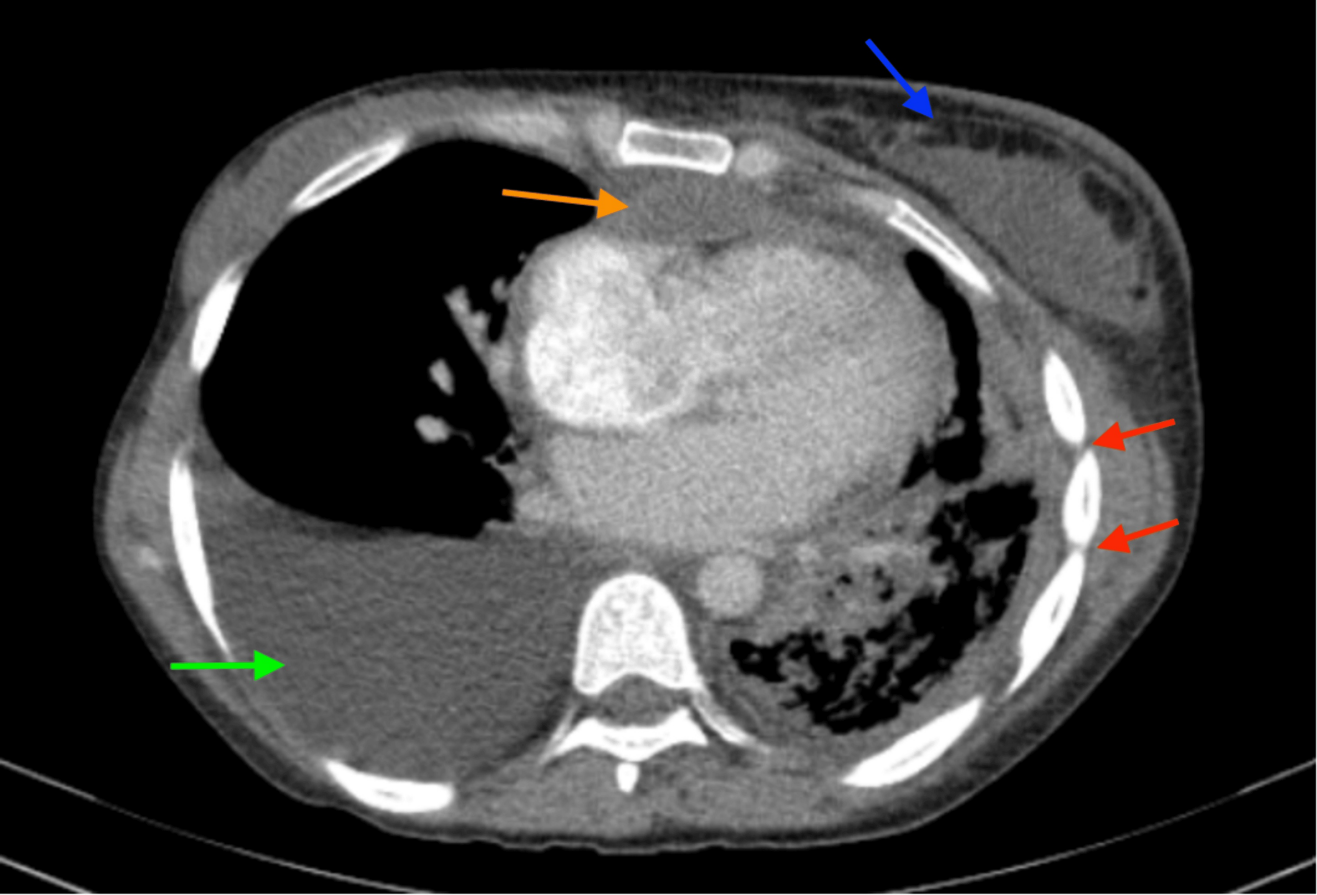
Chest CT showing decreased spacing between ribs on the left (red arrows), a large right-sided pleural effusion (green arrow), pericardial mass (orange arrow), and left breast mass (blue arrow).
